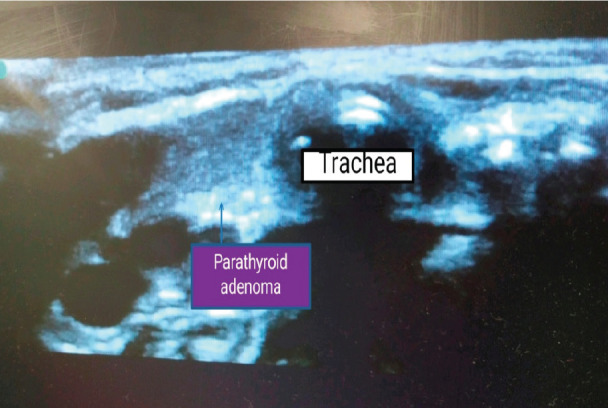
Ultrasound of neck showing parathyroid adenoma
